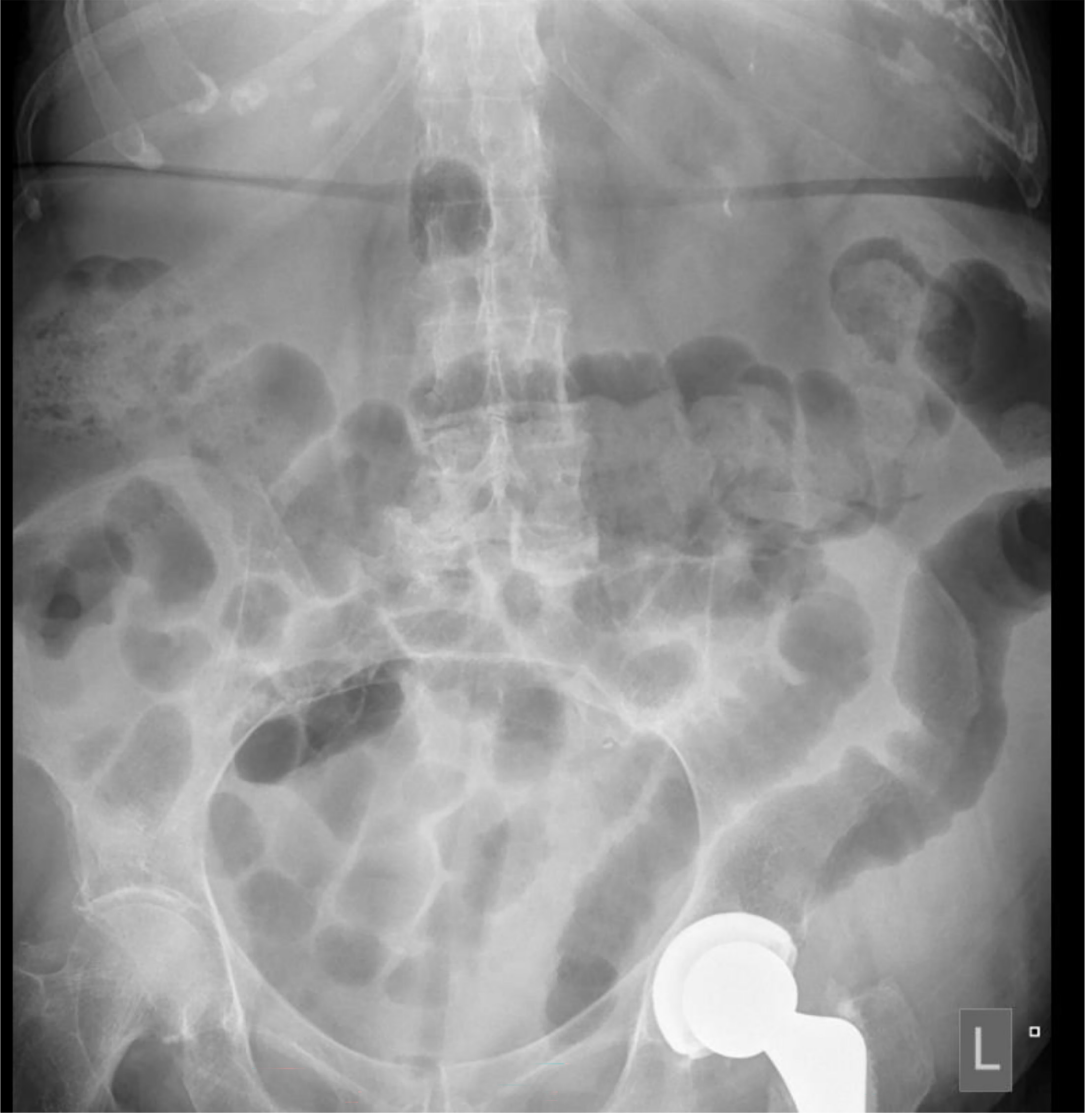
Abdominal X-ray showing evidence of colitis in the descending and sigmoid colon.
